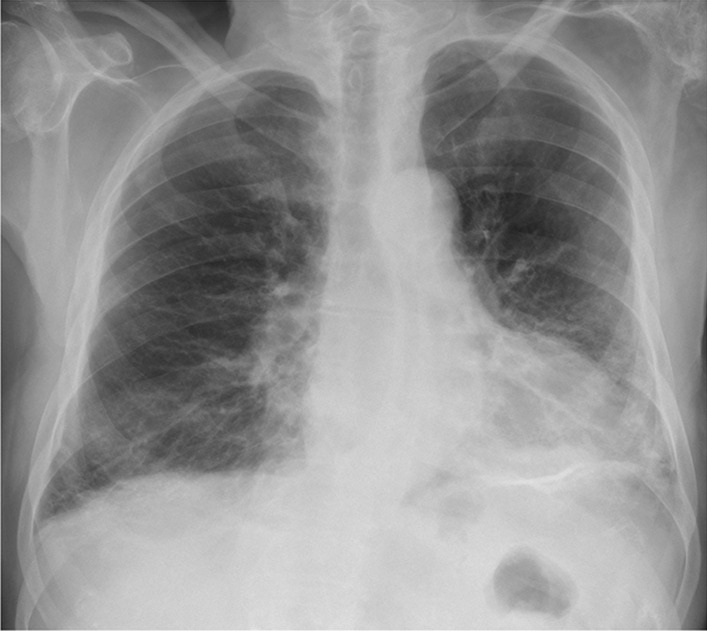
Chest x-ray: bilateral, diffused interstitial opacities in lower lobes, especially by the left side
